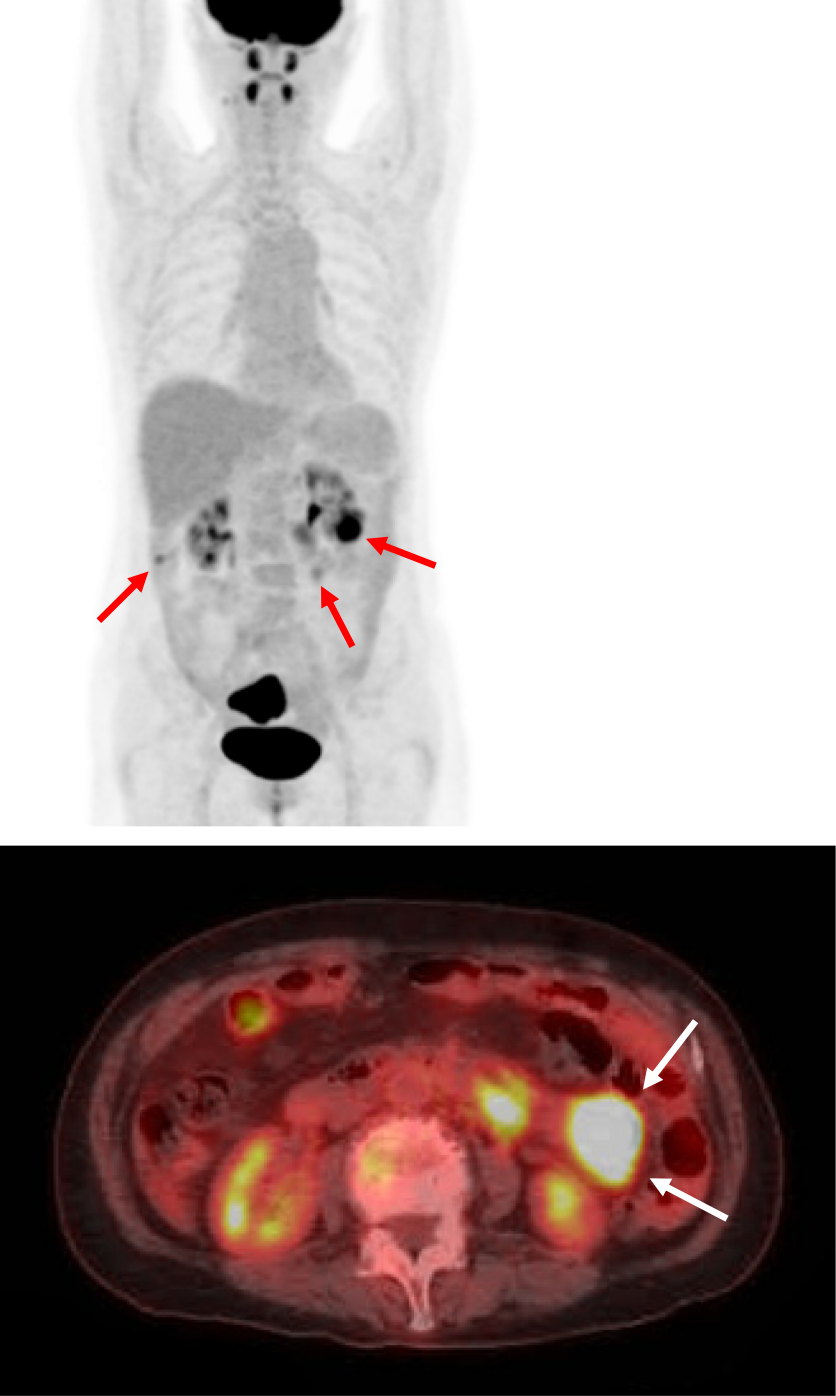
PET-CT shows intense focal FDG uptake in the endometrium and multiple massive lymphadenopathies involving the mesentery and para-aortic region
